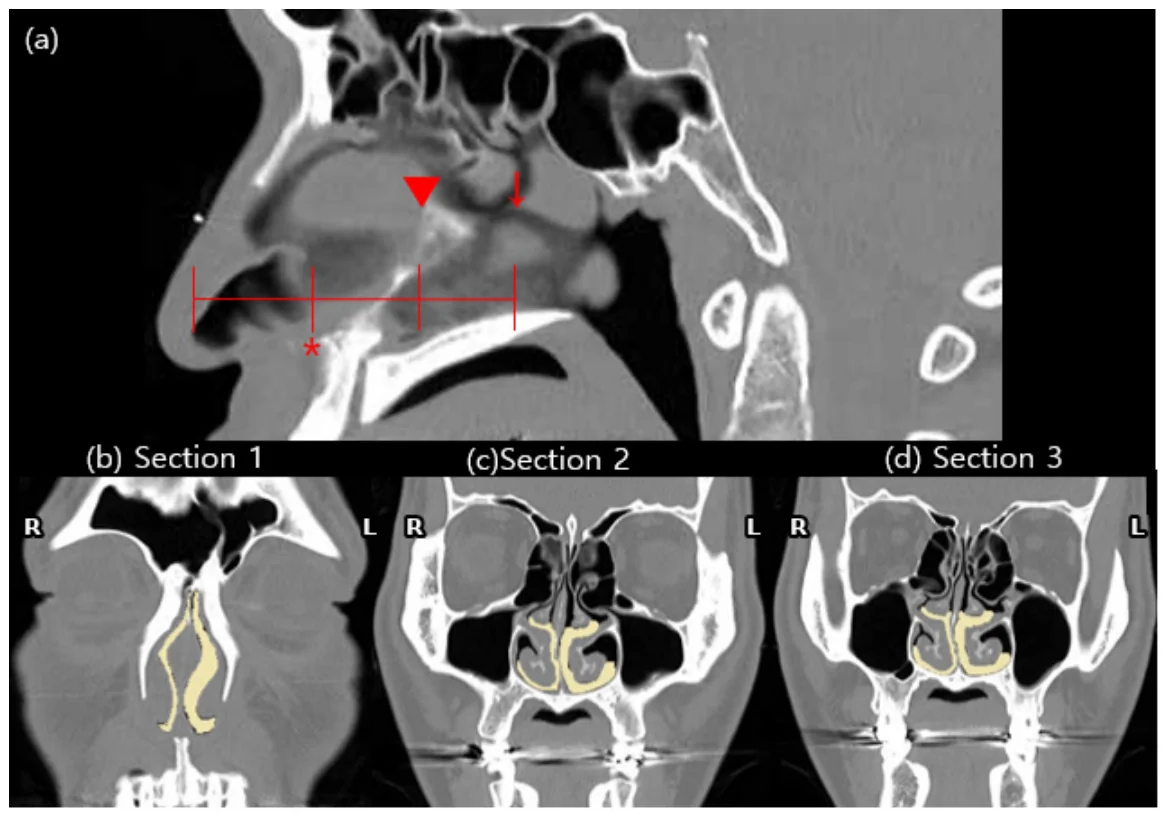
Three distinct sections of the nasal cavity. (a) Sagittal CT view (left side) showing the landmarks that delimit the three sections: the anterior nasal spine of the maxilla (asterisk, *), the junction of the nasal septum (arrowhead, ▼), and the anterior end of the superior turbinate (arrow, ↓). Section 1 extends from the nostril to the anterior nasal spine (*); Section 2, from the anterior nasal spine (*) to the nasal septal junction (▼); and Section 3, from the nasal septal junction (▼) to the anterior end of the superior turbinate (↓). (b) Minimum cross-sectional area (mCSA) in Section 1 (S1). (c) mCSA in Section 2 (S2). (d) mCSA in Section 3 (S3). All measurements were performed in calibrated physical units (mm^2^) on the PACS using DICOM pixel-spacing data and are independent of the displayed image magnification. Window width/level ≈ 2500/600.
